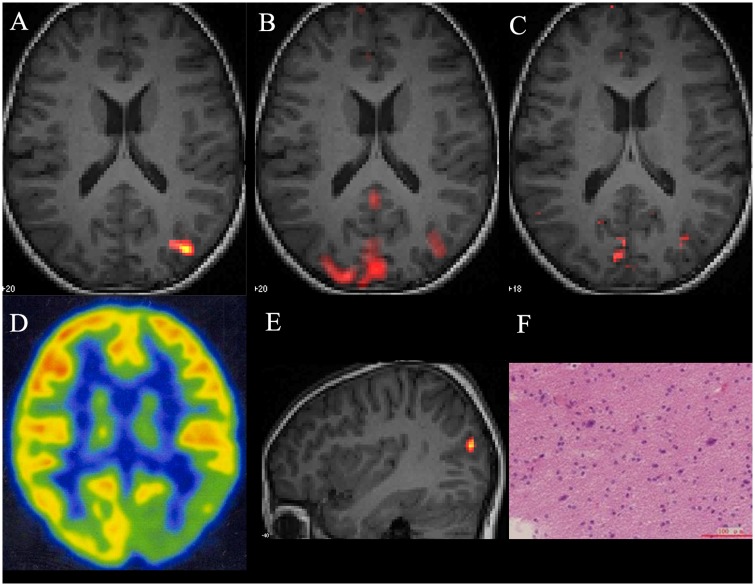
Left parieto-occipital lobe dysplasia in a MRI negative 9-year-old patient. (A) Axial and (E) sagittal fALFF, (B) axial ALFF, and (C) ReHo reveal abnormal activation in the left parieto-occipital lobe. (D) The corresponding ^18^F-FDG PET/CT image shows low FDG uptake in the left frontoparietal region. (F) Pathological confirmation of left parieto-occipital lobe focal cortical dysplasia.
